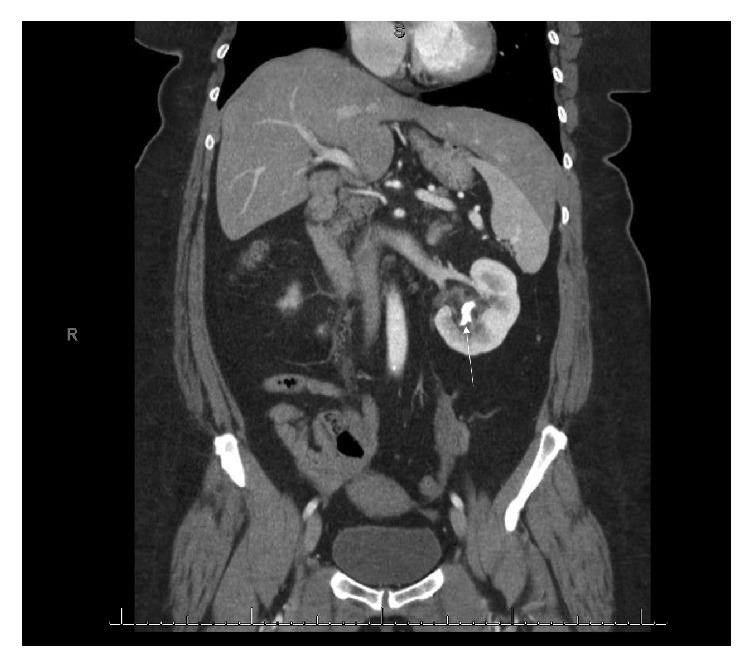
Coronal CT scan of the abdomen and pelvis with contrast revealed a 6 mm obstructing right proximal ureteral stone (not shown in image) causing mild hydronephrosis and a 2 cm left inferior pole staghorn calculus causing calyceal dilatation (arrow).
